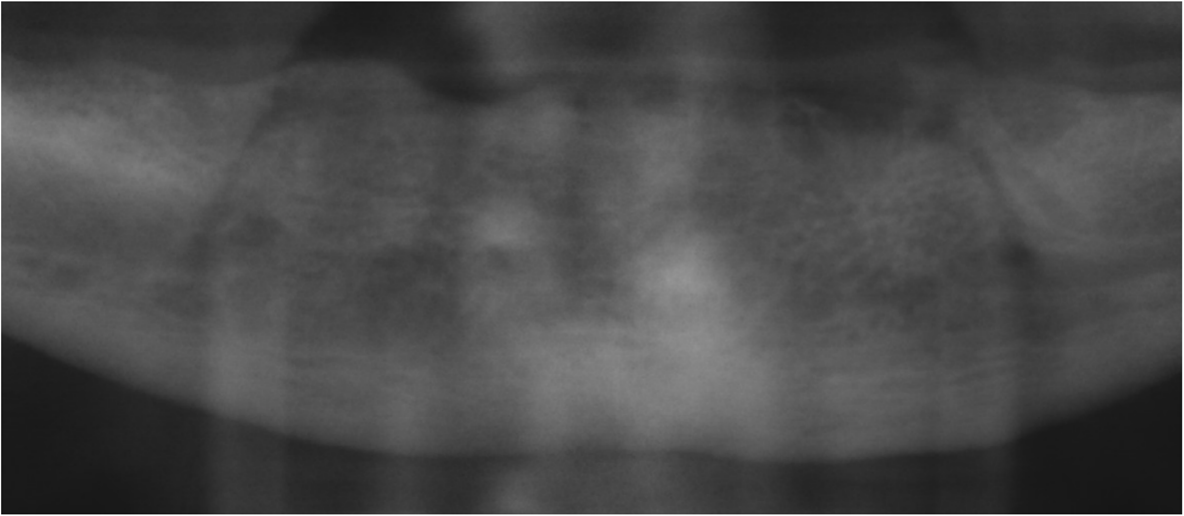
Excerpt from an exemplary preoperative radiography showing the mandibular bone with sufficient height
